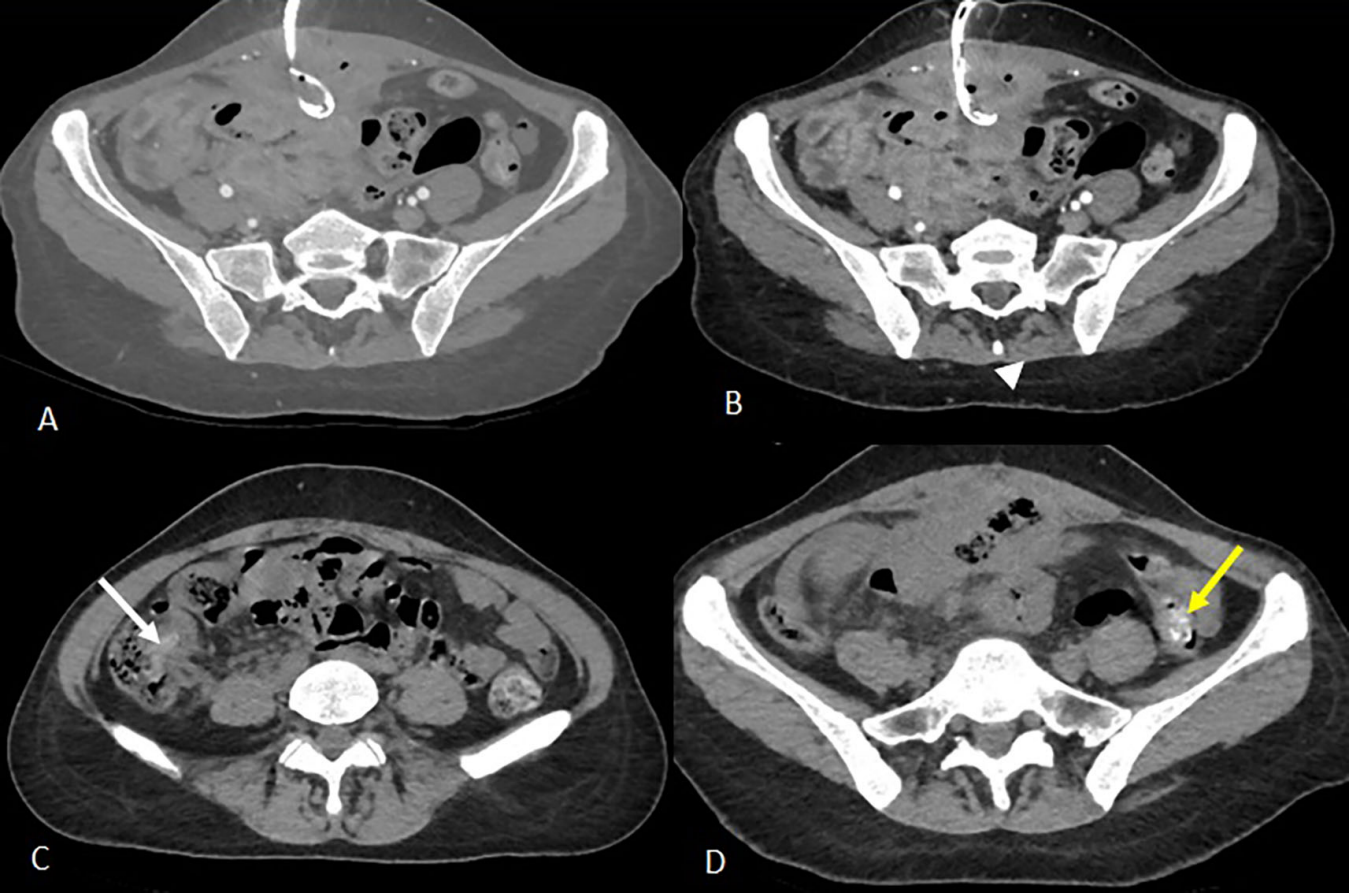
Three days after the first scan, CT-guided drainage of the collection was indicated and performed (
